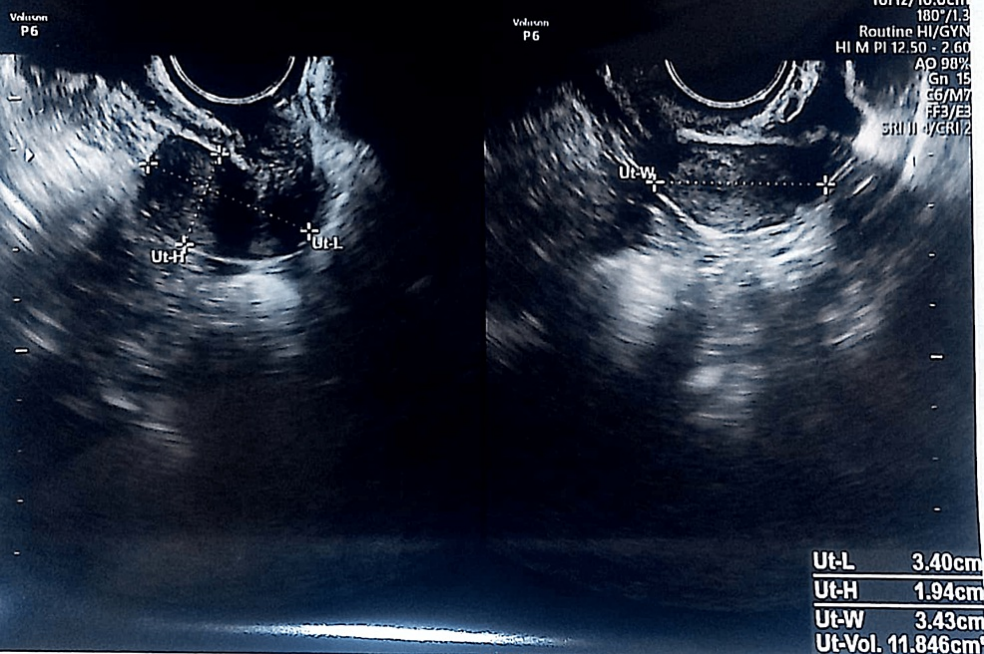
Ultrasound of the uterus
